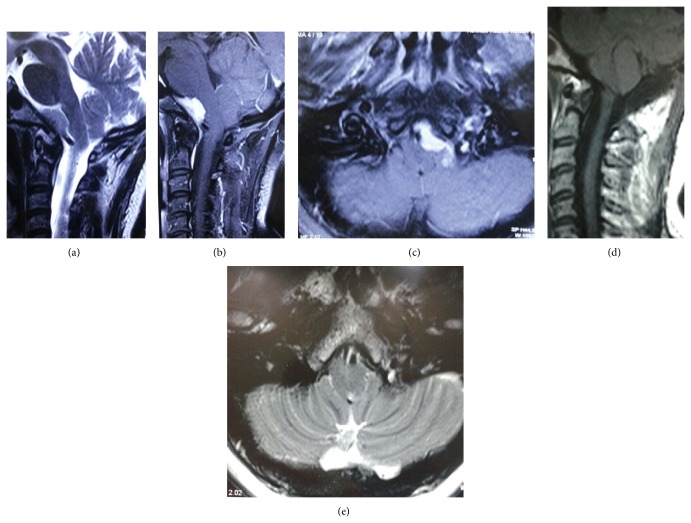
(a) T2 weighted sagittal image; (b) postcontrast T1 weighted sagittal image; (c) postcontrast T1 weighted axial image: magnetic resonance imaging (MRI) showing anterolaterally placed, homogenously enhancing dural based lesion in the foramen magnum encasing the left vertebral artery suggestive of meningioma. (d) T1 weighted sagittal image and (e) T1 weighted axial image: postoperative MRI showing complete excision of tumor.
